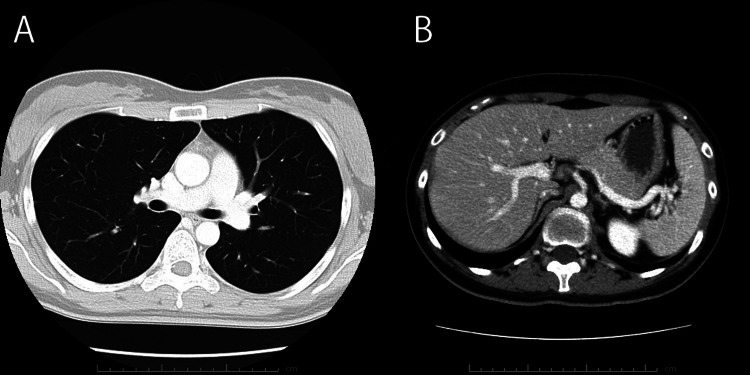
Enhanced chest and abdominal CT images at admission. (A) Chest CT image showing no lung lesions.
